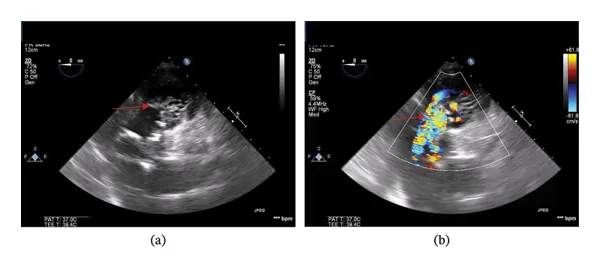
(a) Deep transgastric view demonstrating SAM (red arrow). (b) Deep transgastric view with color Doppler demonstrating flow acceleration through the left ventricular outflow tract (red arrow).
